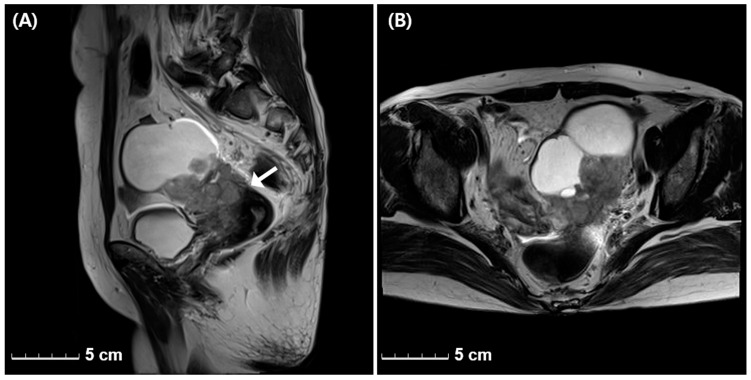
Sagittal (A) and axial (B) T2-weighted pelvic MRI images demonstrate an approximately 10 cm lobulated heterogeneous mass in the left adnexa that is abutting the superior and left lateral aspects of the uterus (arrow).
